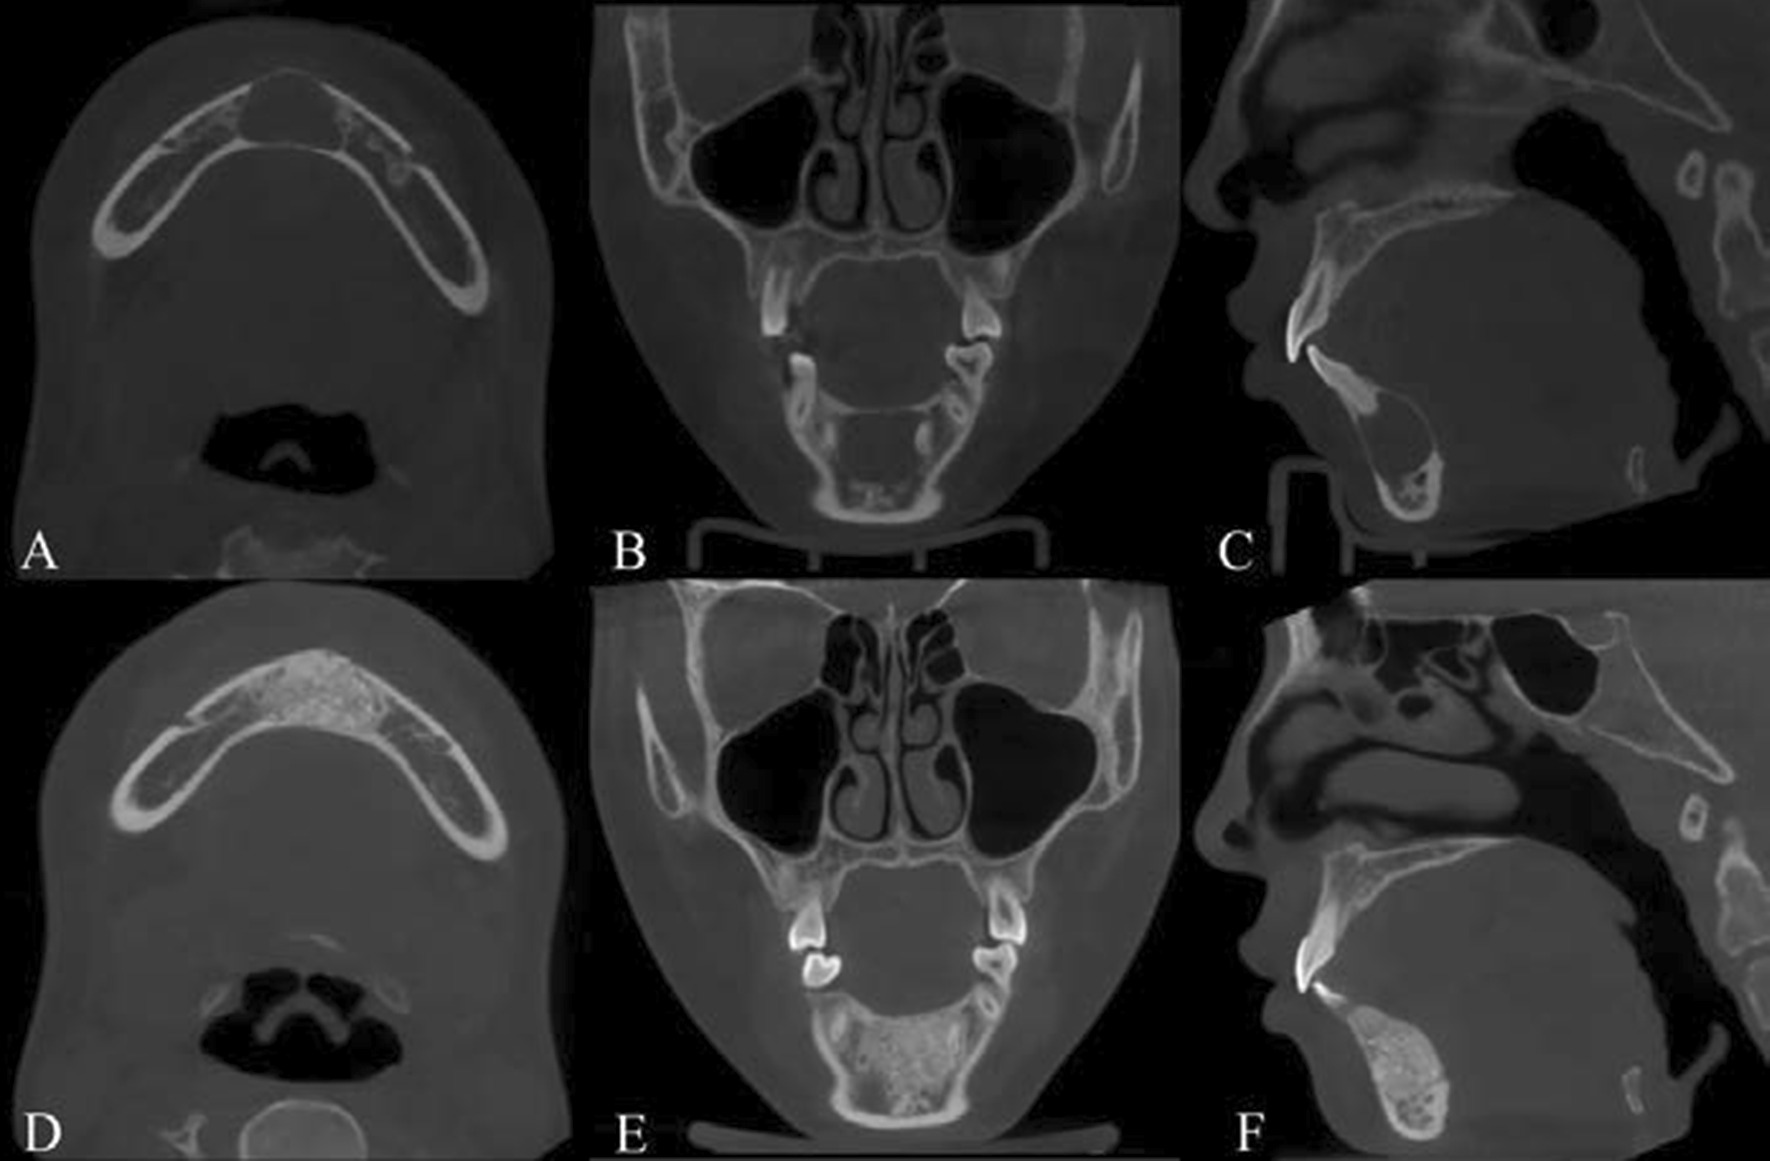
Radiographic features of SBC. A–C CBCT preoperatively for SBC. CBCT shows an isolated, round-like, unilocular radiolucent image of the lower anterior region with no bone-white line and no resorption and displacement of the adjacent root, mild expansion of the buccal cortex and thinning of the buccal and lingual cortex. D–F CBCT postoperation of SBC. CBCT shows dense bone filling within a proto-cyst in the lower anterior region
